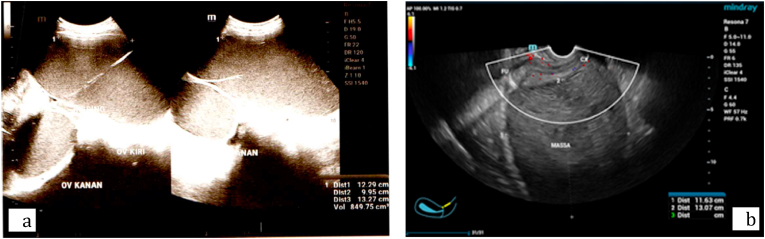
Endometriosis cyst showed on transrectal ultrasound from both patients.
